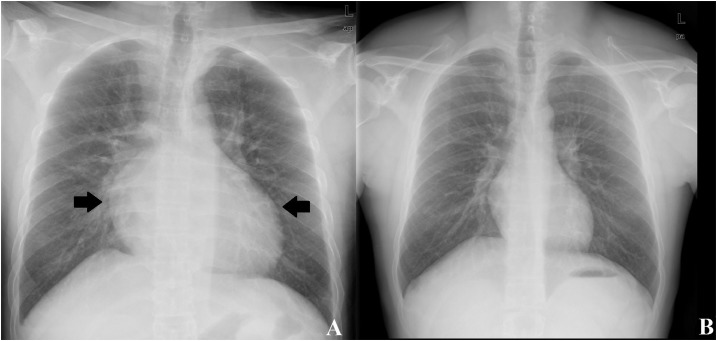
(A) Chest X-ray performed at admission showing severe cardiomegaly; (B) chest X-ray performed 1 year before the admission after a chest trauma which has been used for comparison.
